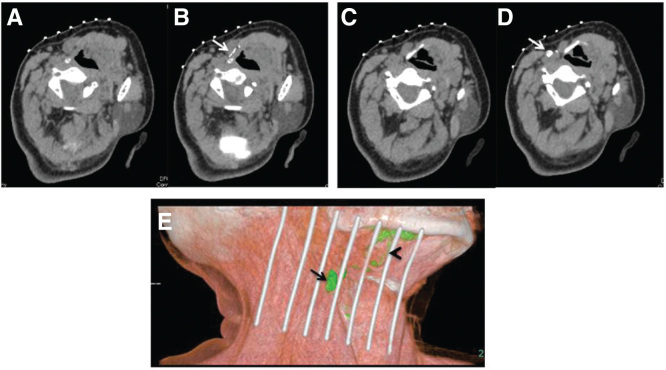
A case of CT lymphography for T2N0 tongue cancer. (A) Control CT image before iopamidol injection (section at lymph vessel level). (B) CT image 1 min after iopamidol injection. Arrow, lymph vessel. (C) Control CT image before iopamidol injection (section at sentinel lymph node (SLN) level). (D) CT image 3 mins after iopamidol injection. Arrow, SLN, which is the first enhanced lymph node. (E) Three-dimensional CT lymphography reconstructed from the images after injection. Arrow, SLN; arrowhead, lymph vessel.
